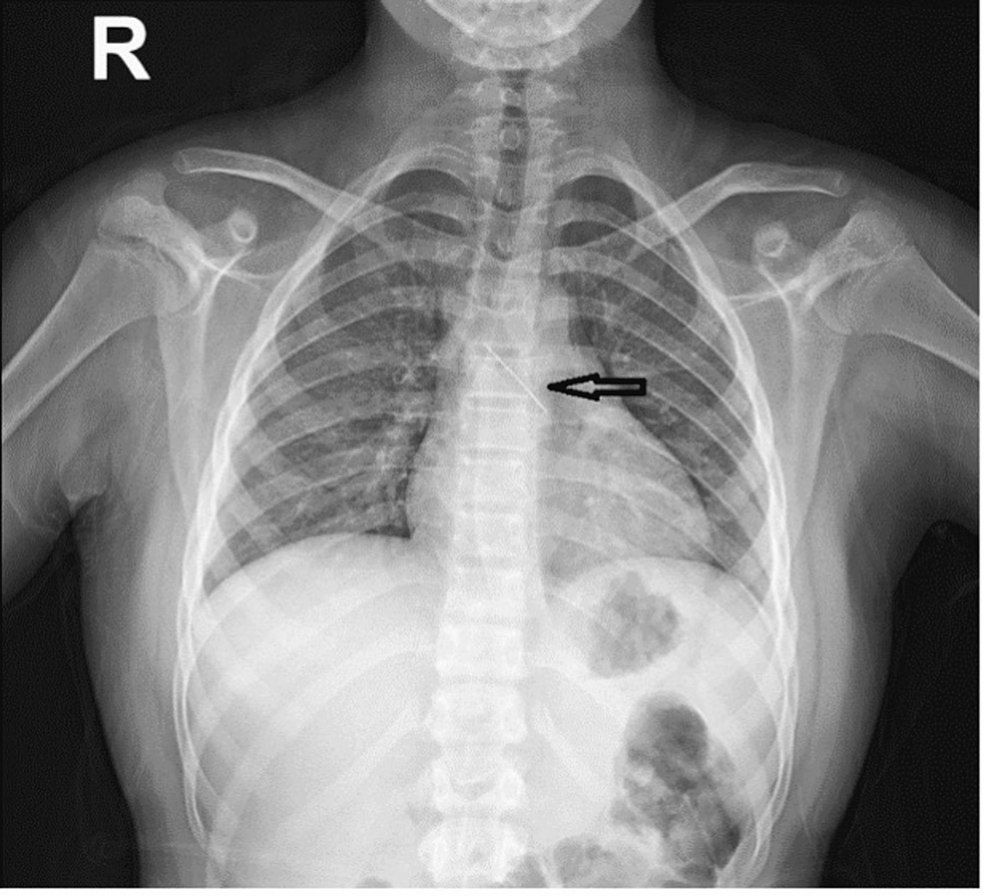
Chest radiography of one of the patients before bronchoscopy - turban pin is in the left main bronchus
